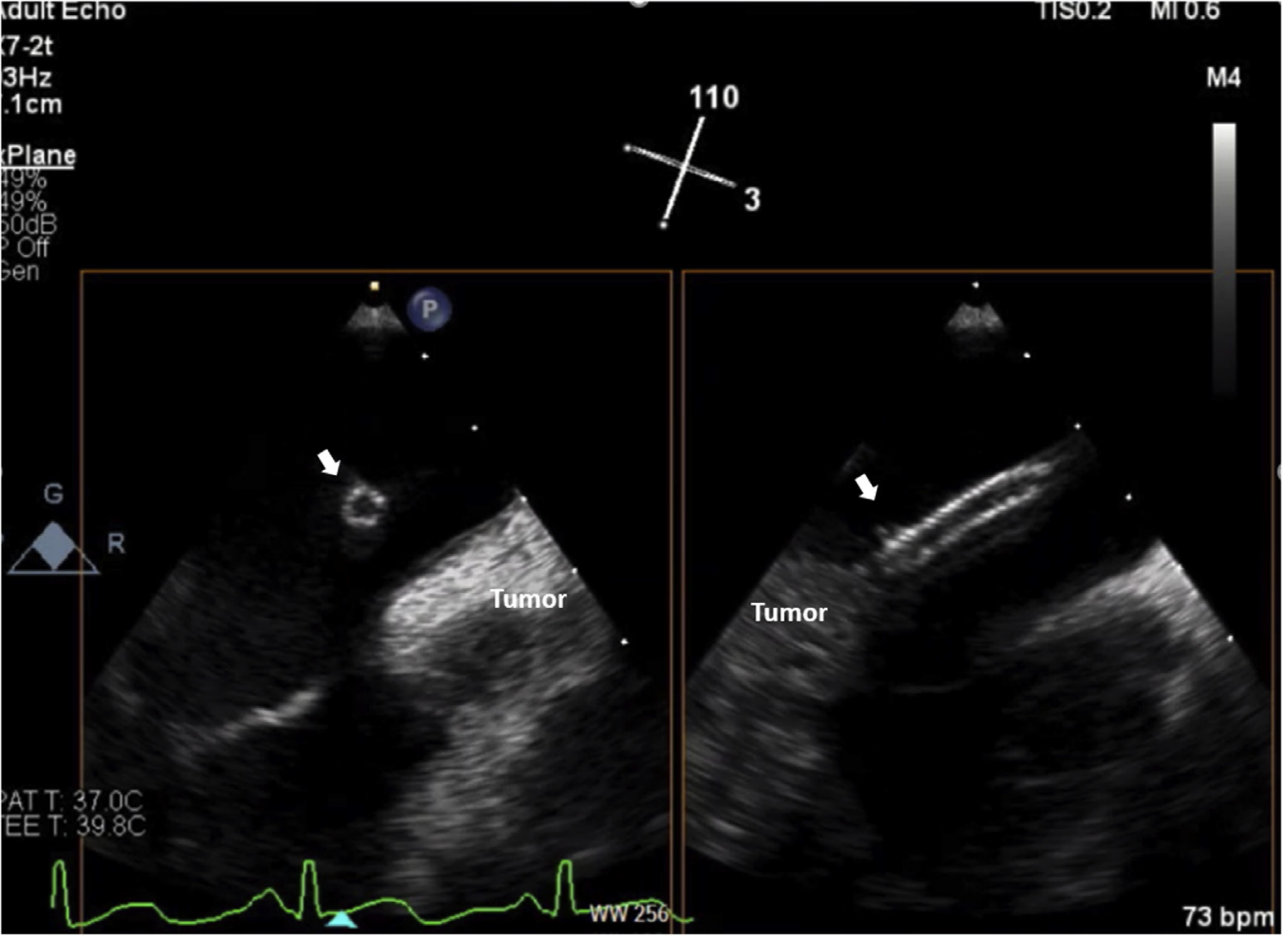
Transesophageal Echocardiography With Biplane Imaging During Endomyocardial Biopsy The image shows the biopsy forceps (arrows) directed against the cardiac mass and sampling the mass.
